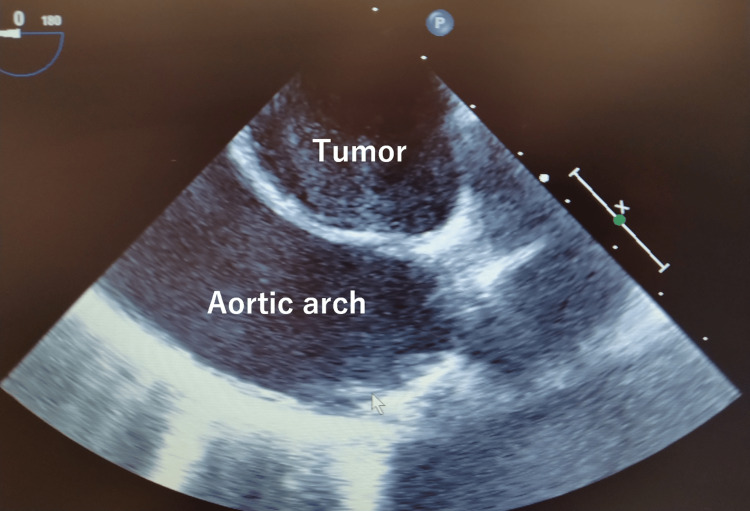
TEE showing that the tumor was mobile and did not invade the aortic arch (after induction of anesthesia) TEE, transesophageal echocardiography
